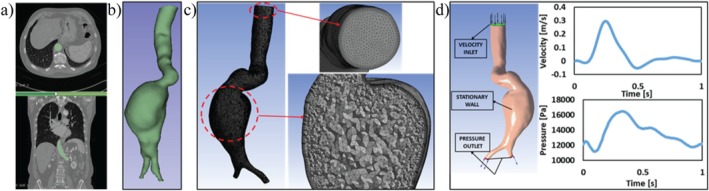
CFD model generation: (a) Patient‐specific CT images. (b) 3D geometry segmentation by using 3D Slicer software. (c) Generated a tetrahedral mesh with inflation layers on the boundaries. (d) Transient CFD analysis boundary condition locations on the AAA model, and Velocity–Time and Pressure–Time graphs used as inlet and outlet conditions [28] (Reprinted with permission).
